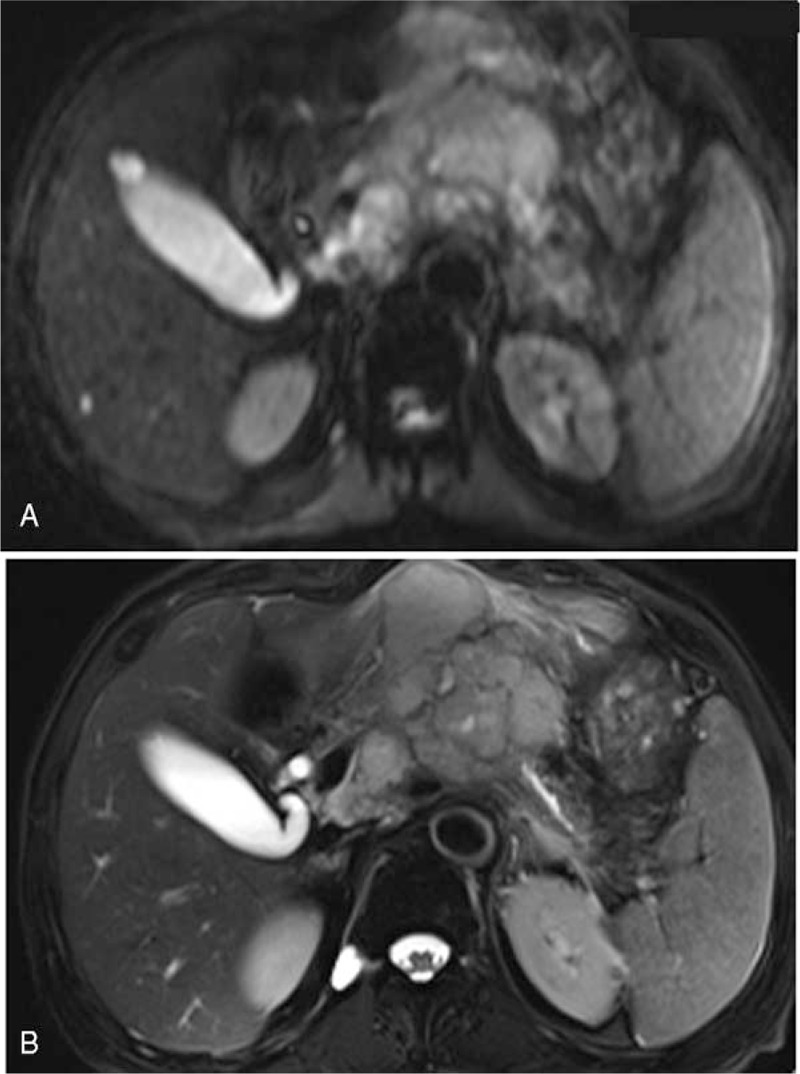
Preoperative magnetic resonance images of the patient. A. The lesion in the pancreatic body has high signal intensity on diffusion-weighted imaging. B. On T2-weighted images, the mass exhibits high signal intensity.
